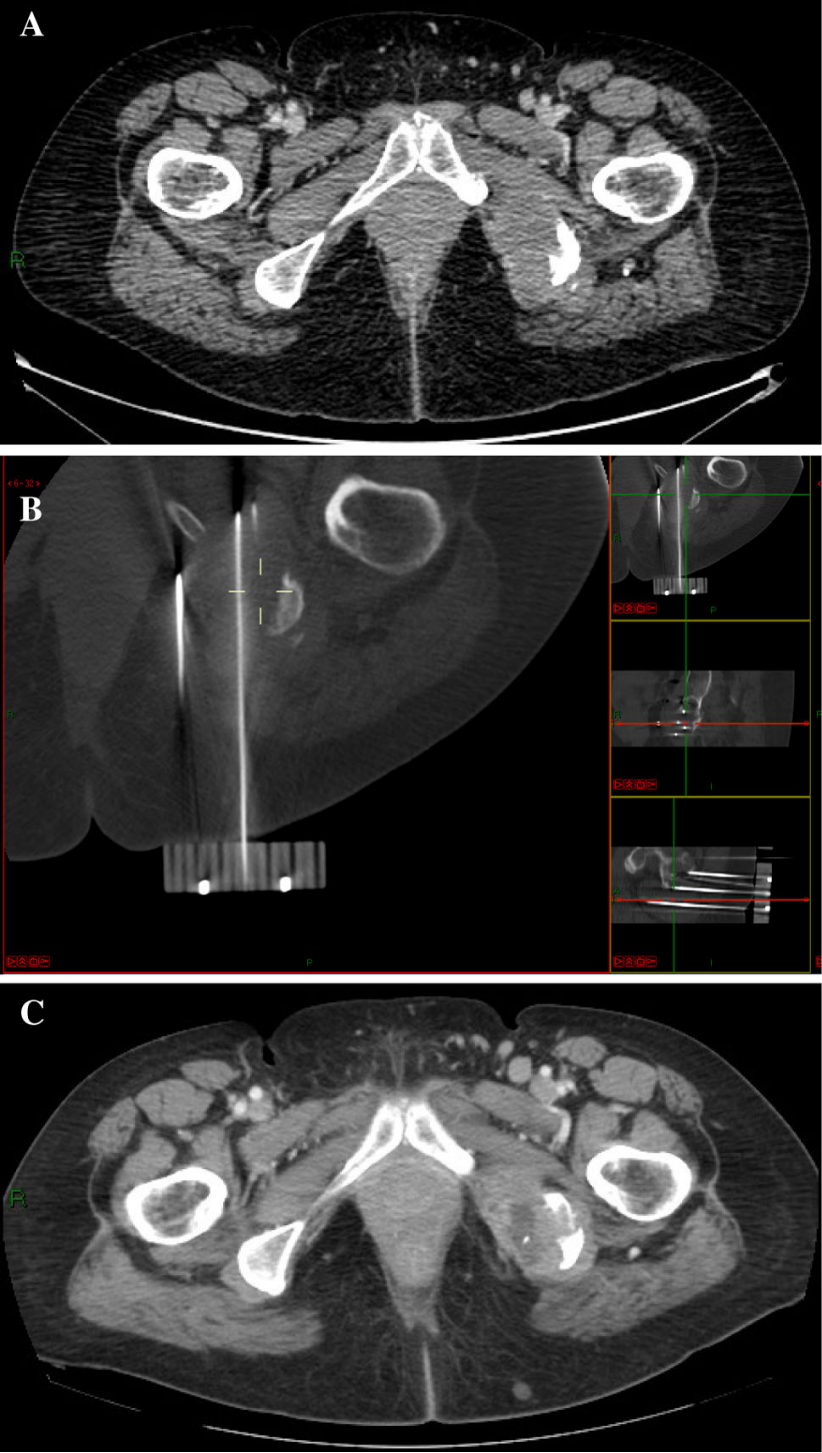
a–c A 57-year-old patient: left ischium painful bone metastases, kidney carcinoma, a CT-guided ECT needles positioning and multiplanar reconstruction, b CT scan at 7 months follow-up; central necrosis and shrinking of the metastatic lesion: Partial Response (c)
